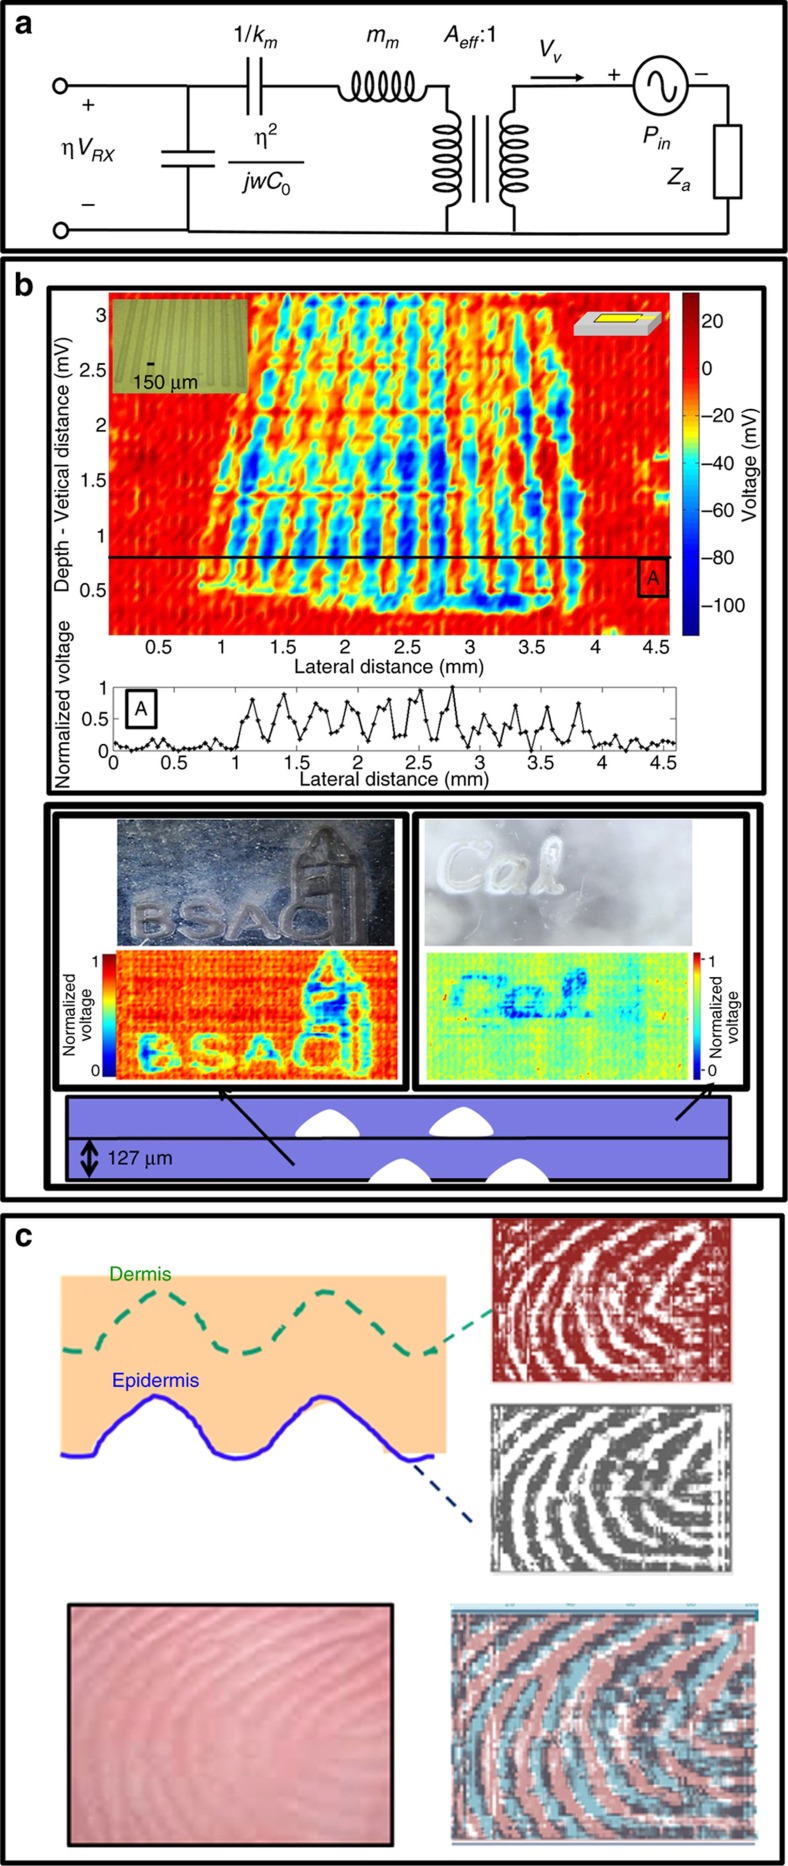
(a) Equivalent circuit model for receiving. (b) Pulse-echo images. Top: National Institute of Standards and Technology fingerprint resolution test composed of converging parallel lines. Bottom: axial resolution demonstration using two overlaid patterns separated by 127 μm in depth. (c) Ultrasound fingerprint image collected at the epidermis and sub-epidermis layer (top). Good agreement is observed between the ultrasonic images and the optical fingerprint image.
